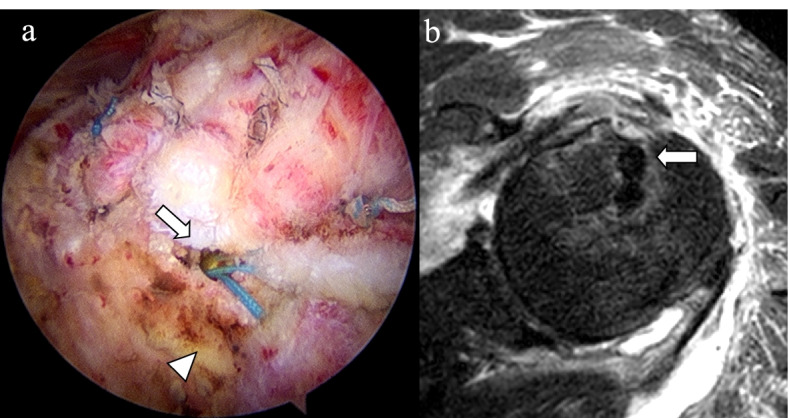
a Arthroscopic RCR was performed. A 2.8 mm all-suture anchor was inserted to the upper part of the intertubercular groove (arrow), and to examine the anchor settling, the area surrounding the anchor was given the debridement using radiofrequency (arrowhead); b the insertion of the anchor to the intertubercular groove is shown on the immediate post-operative MRI (arrow)
